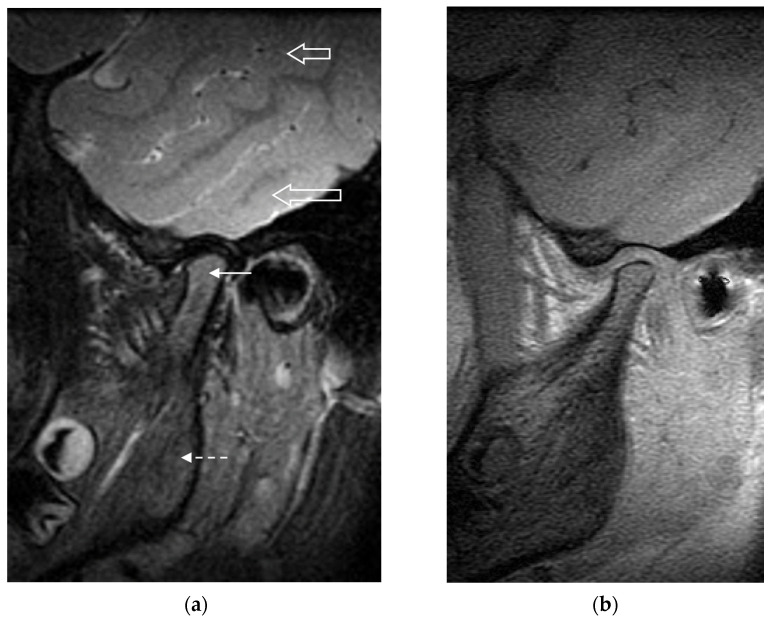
(a) Sagittal T2-weighted fat suppressed MR image of the left temporomandibular joint in a 9-year-old girl. The signal intensity of the bone marrow in the mandibular condyle (solid arrow) is increased compared to the signal intensity of that in the mandibular ramus (dashed arrow). Following the exact definition of bone marrow edema in the scoring system would cause this to be scored as grade 1 (present) bone marrow edema. However, this increased signal intensity is likely secondary to the generally higher signal in structures at the center of the field of view versus those at the periphery: notice the higher signal intensity of brain parenchyma nearer the center of the image (lower open arrow) versus that at the periphery of the image (upper open arrow). If this were true marrow edema, the signal intensity on the corresponding precontrast T1-weighted image (b) would be expected to be decreased, which was not the case.
